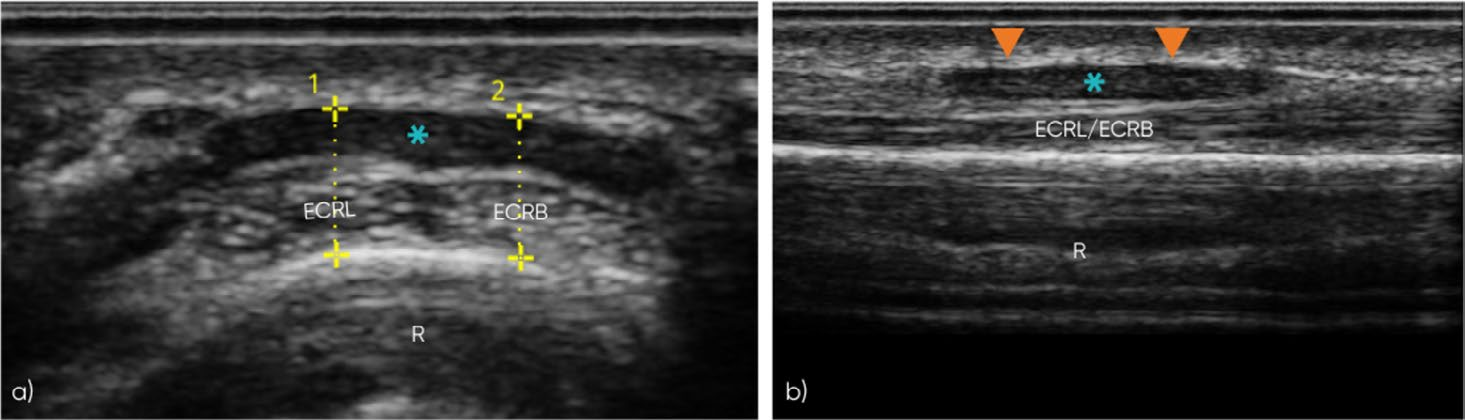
Ultrasound assessment of the symptomatic limb, at the proximal intersection, post‐treatment. (a) Short‐axis ultrasound image of the distal site of the proximal intersection demonstrating normal tendon echogenicity and thickness post‐treatment, with measurements of 3.83 mm (ECRL‐EPB) and 3.72 mm (ECRB‐EPB). No anechoic fluid or signs of hyperemia were detected. (b) Long‐axis ultrasound image of the EPB muscle at the same site demonstrating persistent hypertrophy of the muscle belly, which occupied considerable space above the second compartment. The muscle exhibited normal echogenicity, and the superficial connective tissue sheath showed comparable thickness to the contralateral limb, indicating no evidence of muscle edema. *R*, radius; ECRL, extensor carpi radialis longus tendon; ECRB, extensor carpi radialis brevis tendon; blue asterisk, extensor pollicis brevis muscle; orange arrowheads, superficial connective tissue/fascia; yellow dotted lines with numbers, measurement sites for tendon thickness recorded during ultrasound scanning.
